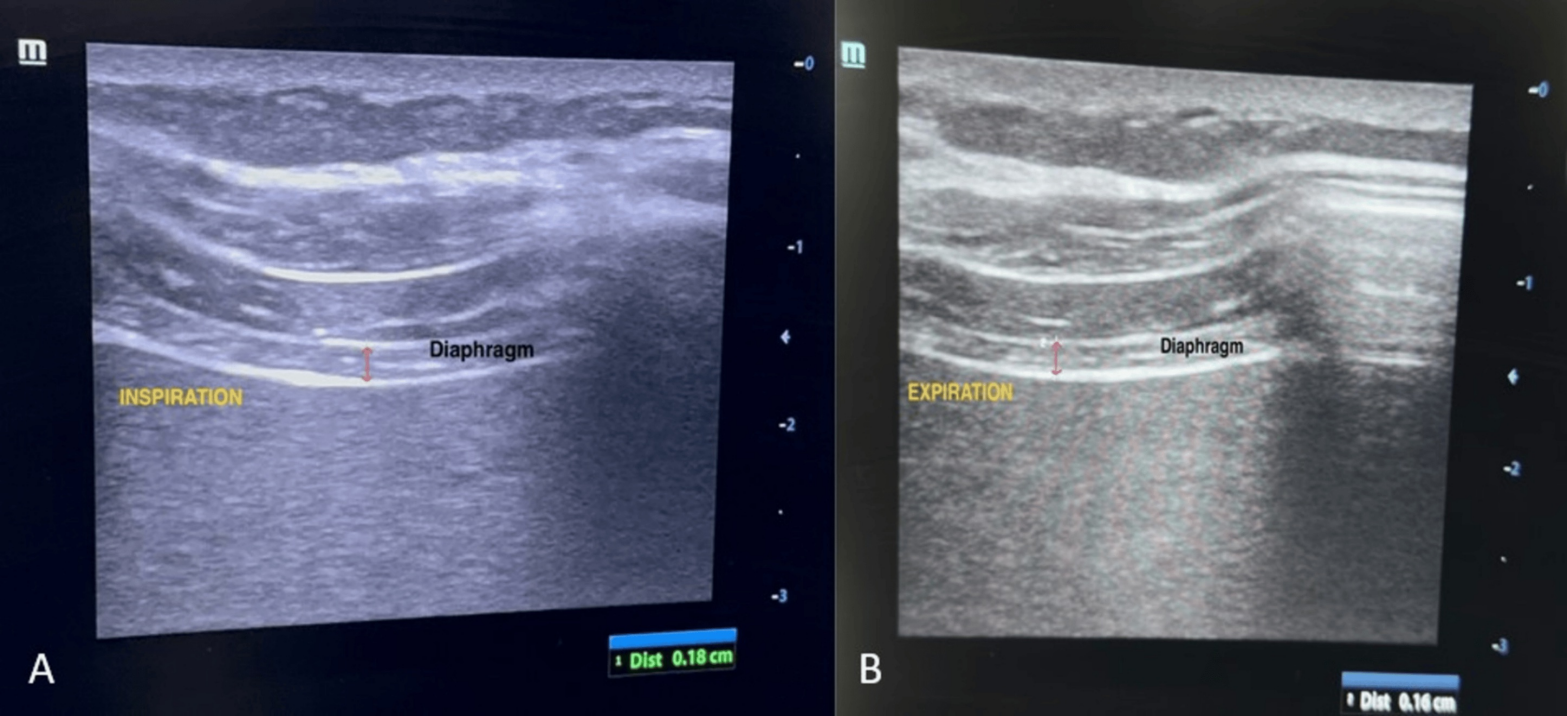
Right diaphragm thickness measured using B-mode sonography with the linear probe at end-inspiration (A) and end-expiration (B).
